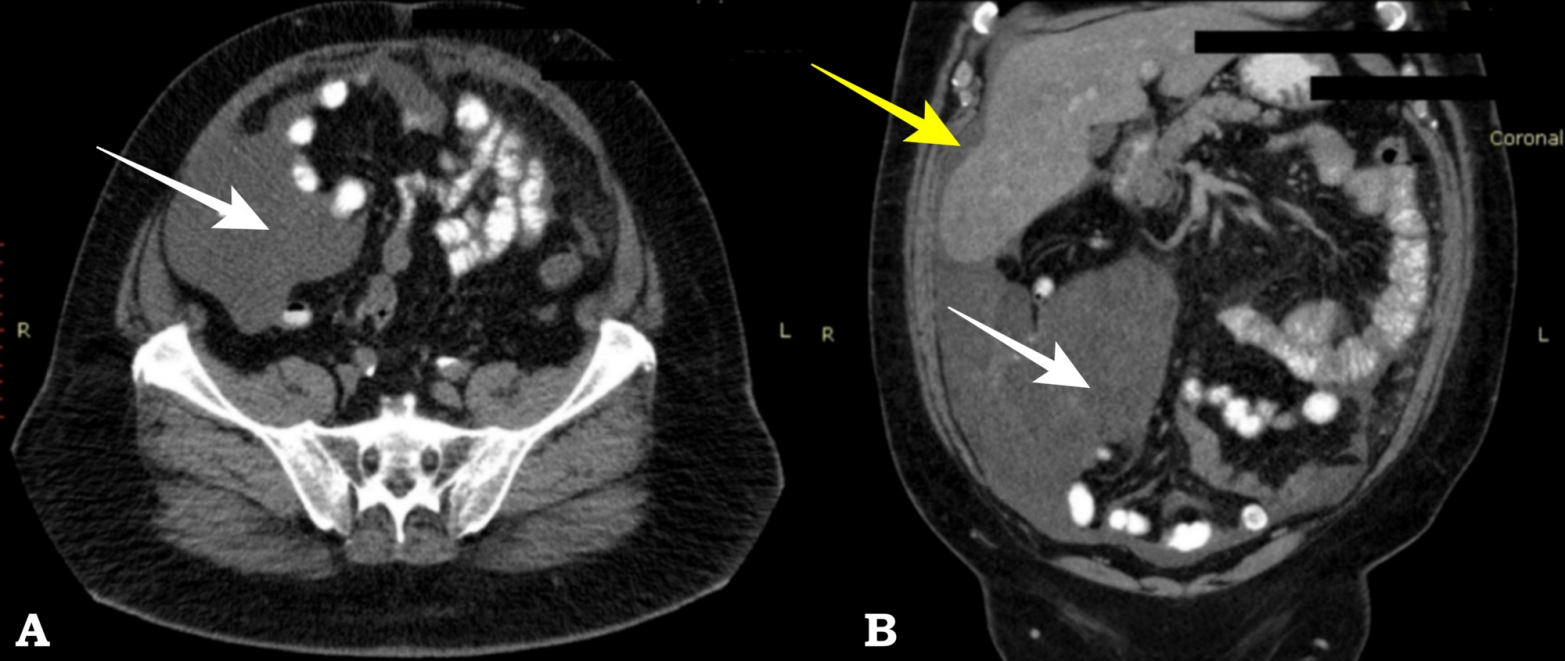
Pseudomyxoma peritonei, (patient #2) Axial computed tomography (CT) (A) and coronal CT reconstruction (B) show extensive low density tissue involving the right lower abdominal quadrant (white arrow) and perihepatic space (yellow arrow)
